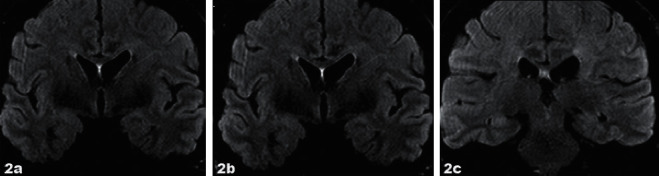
(a–c) Coronal FLAIR-MRI scan after treatment, four weeks later, was normal.
